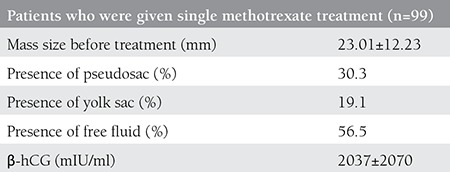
Transvaginal ultrasonography and laboratory results of patients who were given single-dose methotrexate treatment
